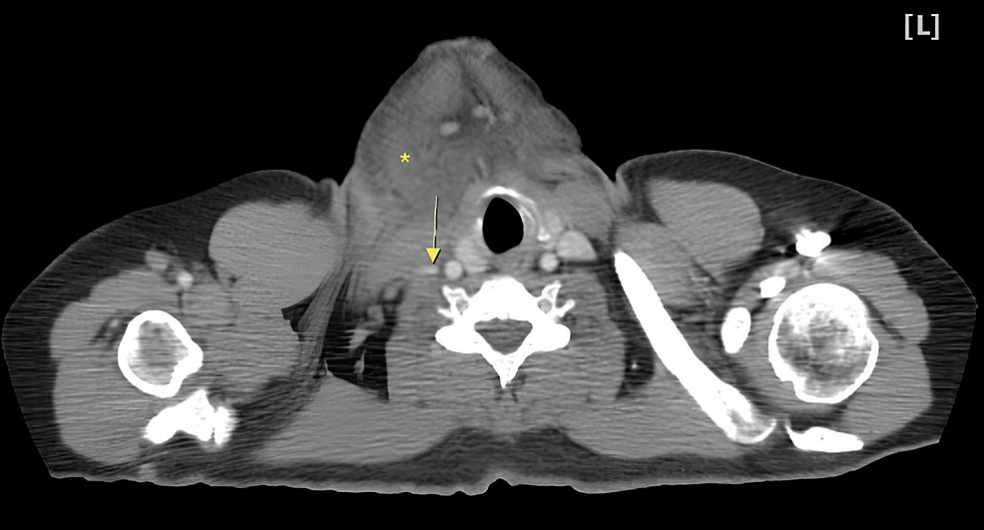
The computed tomography of the neck showed right neck swelling and reduced compressive blood flow, thrombophlebitis and a small amount of thrombus found in the right internal jugular vein.
